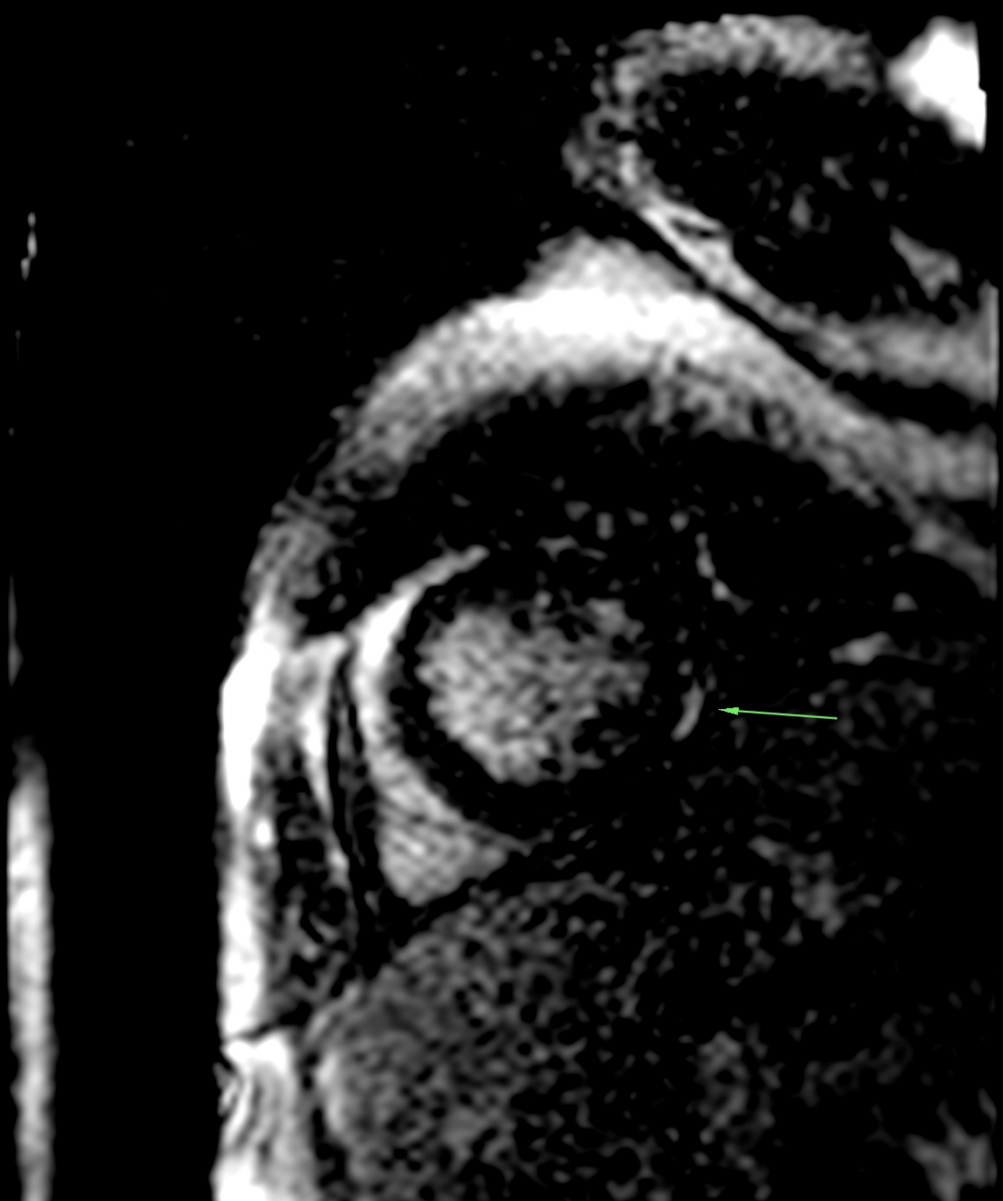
STIR MRI sequence in a short-axis view showing late subepicardial enhancement of the lateral wall of the left ventricle (LV) suggesting myocardial oedema in these areas (indicated by green arrow). STIR MRI: short-tau inversion recovery magnetic resonance imaging
